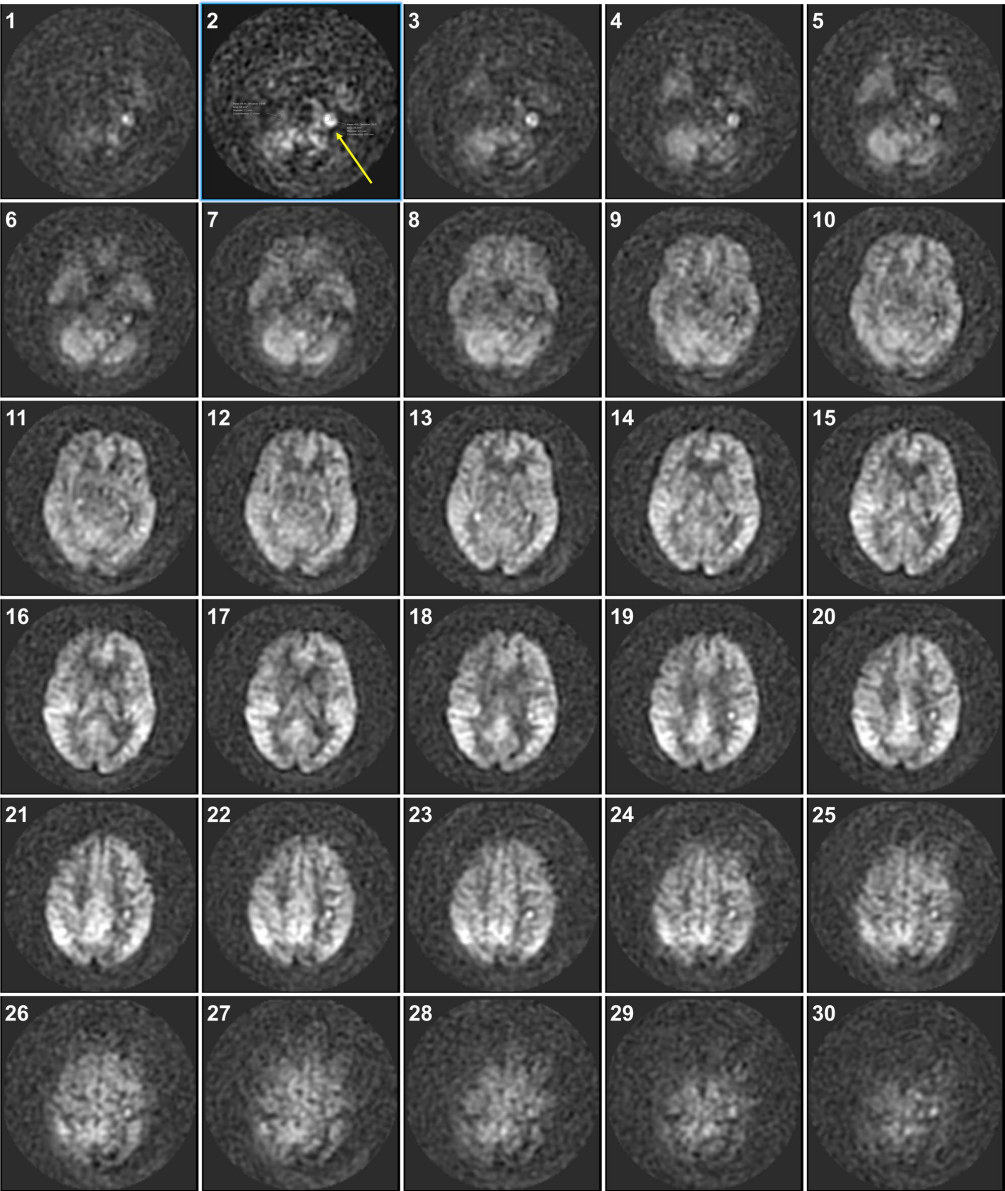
Appearances of all axial slices for an ASL-MRI study on an 80-year-old female showing ASL hyperintensity at the left skull base. Caudad images through the skull base start at image 1 and successive craniad sections end at the vertex at image 30. Successive images 1 to 6 show a focal left-sided skull base hyperintensity with the strongest signal seen on image 2 (arrow).
